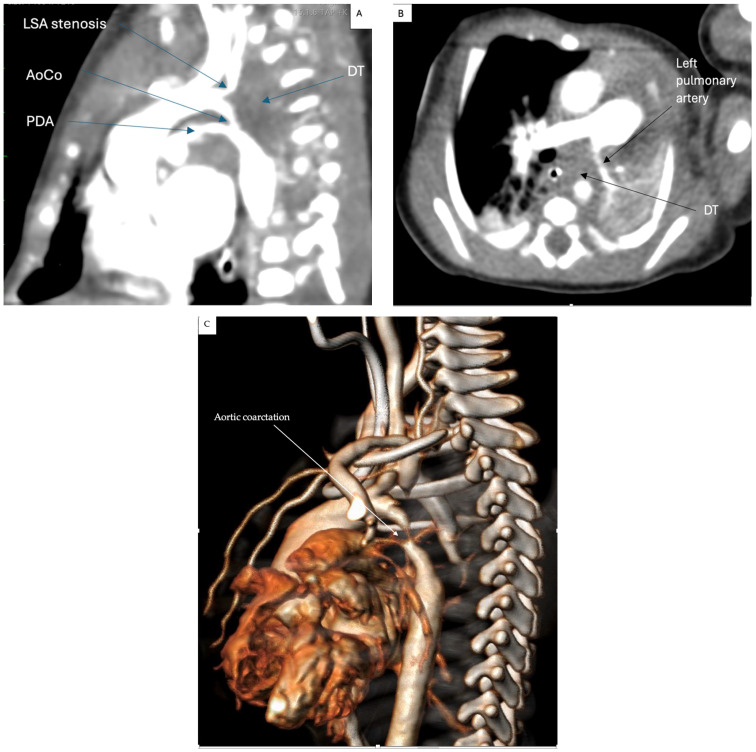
Chest CT angiography. (A) LSA: Left subclavian artery, proximal stenosis; CoAo: aortic coarctation; PDA: persistent ductus arteriosus; DT: desmoid tumor; (B) desmoid tumor (DT) compressing the left pulmonary artery and invasion of the left main bronchus which cannot be visualized; (C) 3D CT reconstruction.
